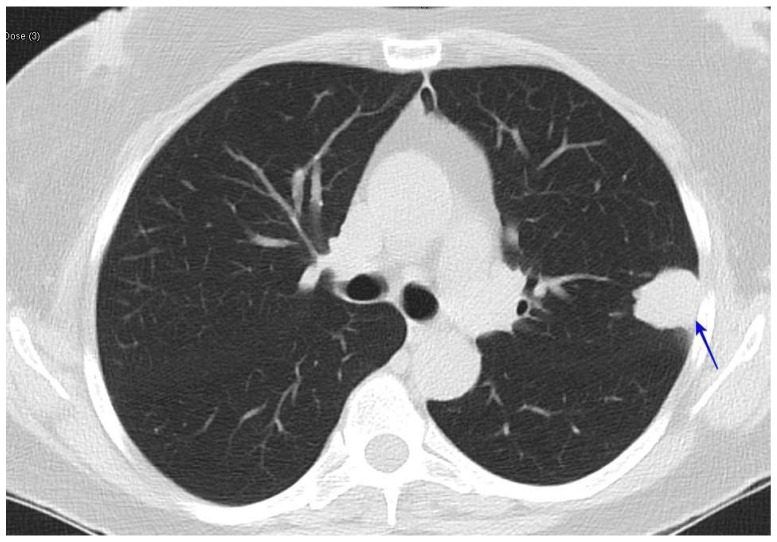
A CT scan with a left upper lobe nodule (blue arrow). The patient was a 64-year-old woman who presented with an incidental 2.5 cm left upper lobe nodule. She underwent a bronchoscopy with a biopsy, which was not diagnostic. Then, she underwent a CT guided biopsy, which showed necrotizing granuloma with fungal spherules consistent with coccidioidomycosis.
